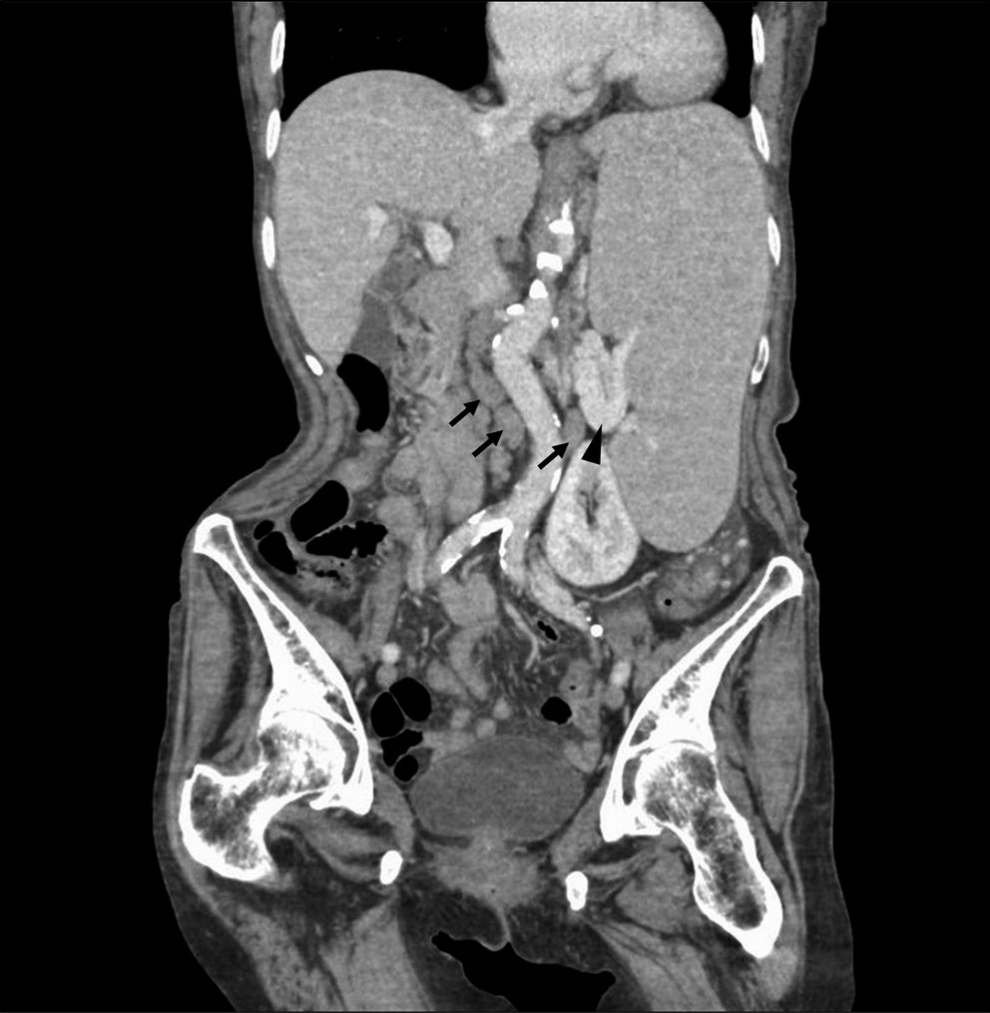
Abdominal contrast‐enhanced computed tomography findings. Abdominal contrast‐enhanced computed tomography images showing massive splenomegaly with a dilated splenic vein (arrowhead) and enlarged para‐aortic lymph nodes (arrows)
